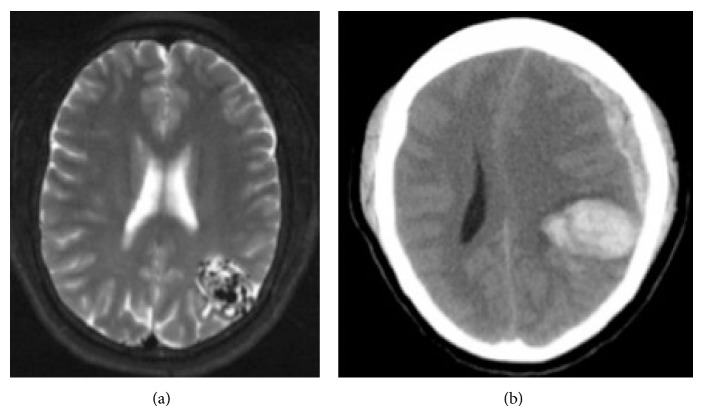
(a) Axial T2 MRI demonstrating left parietal AVM extending from the posterior horn of the left lateral ventricle to the cortex of the left parietal lobe; (b) axial CT demonstrating left parietal ICH and frontoparietal SDH. There is approximately 1.2 cm of left-to-right midline shift.
